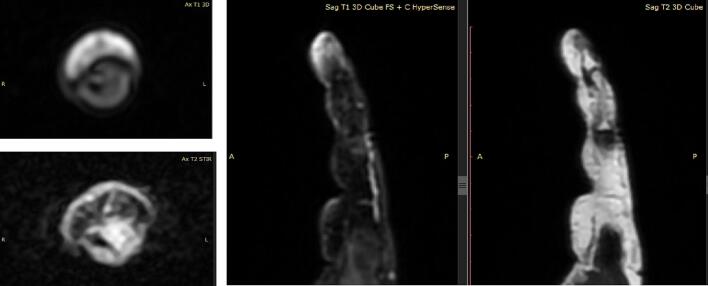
On MRI examination, the lesions with an erosion of the head of the distal phalanx of the middle finger.
